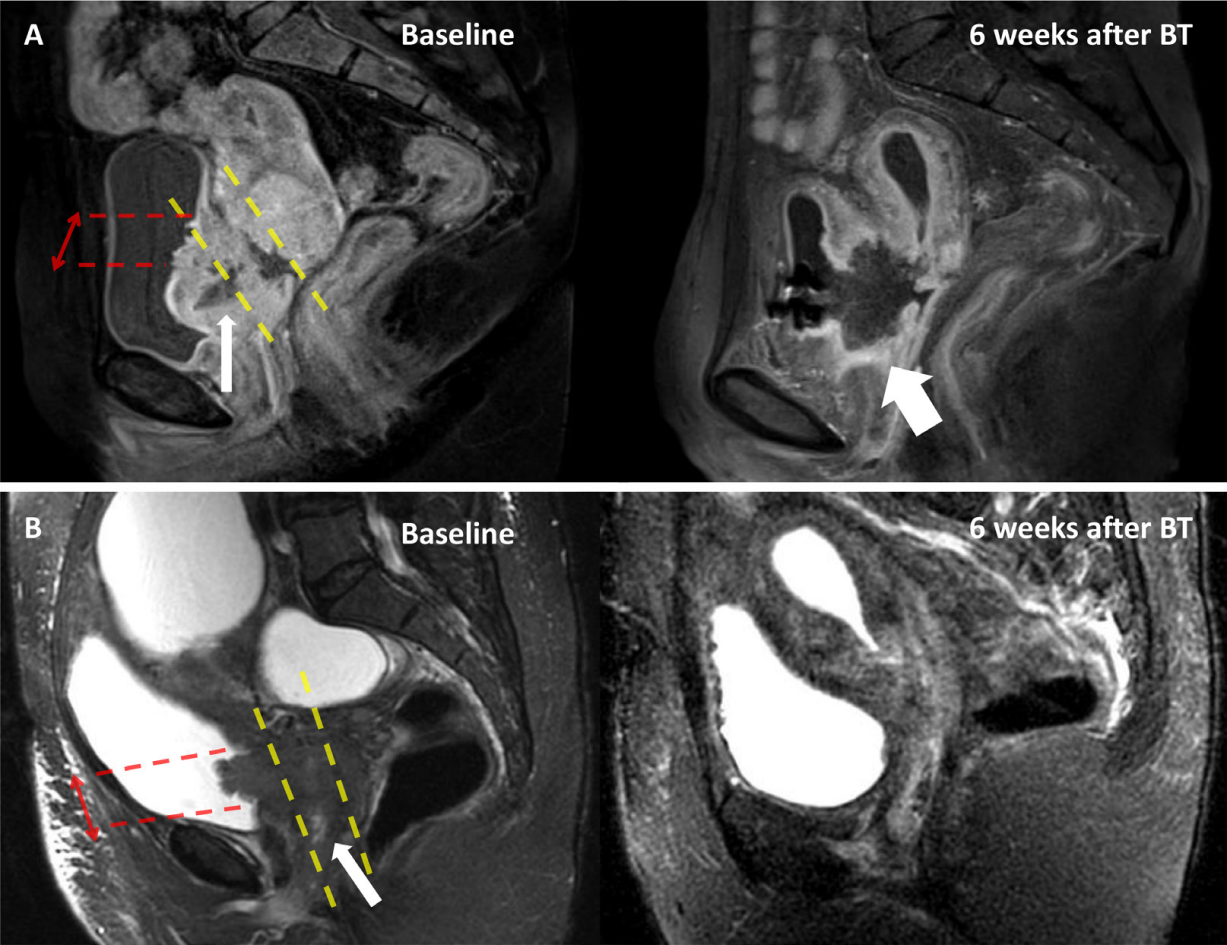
Example of anterior necrosis leading to VVF Magnetic Resonance Imaging (MRI) exams of two patients with cervical cancer stage IVA (patient A: contrast enhanced T1-weighted MRI and patient B: T2-weighted MRI) at baseline (left), and 6 weeks after brachytherapy (right). Patient A had a tumor necrosis (thin arrow) which involved the anterior third of the tumor, while there was no anterior necrosis for patient B. Six weeks after brachytherapy (BT), patient A developed a vesicovaginal fistula (thick arrow), while patient B had a complete response. Red arrows: height of the bladder involvement. Yellow dashed line: delimit the anterior-third, mid-third and posterior-third of the tumor.
